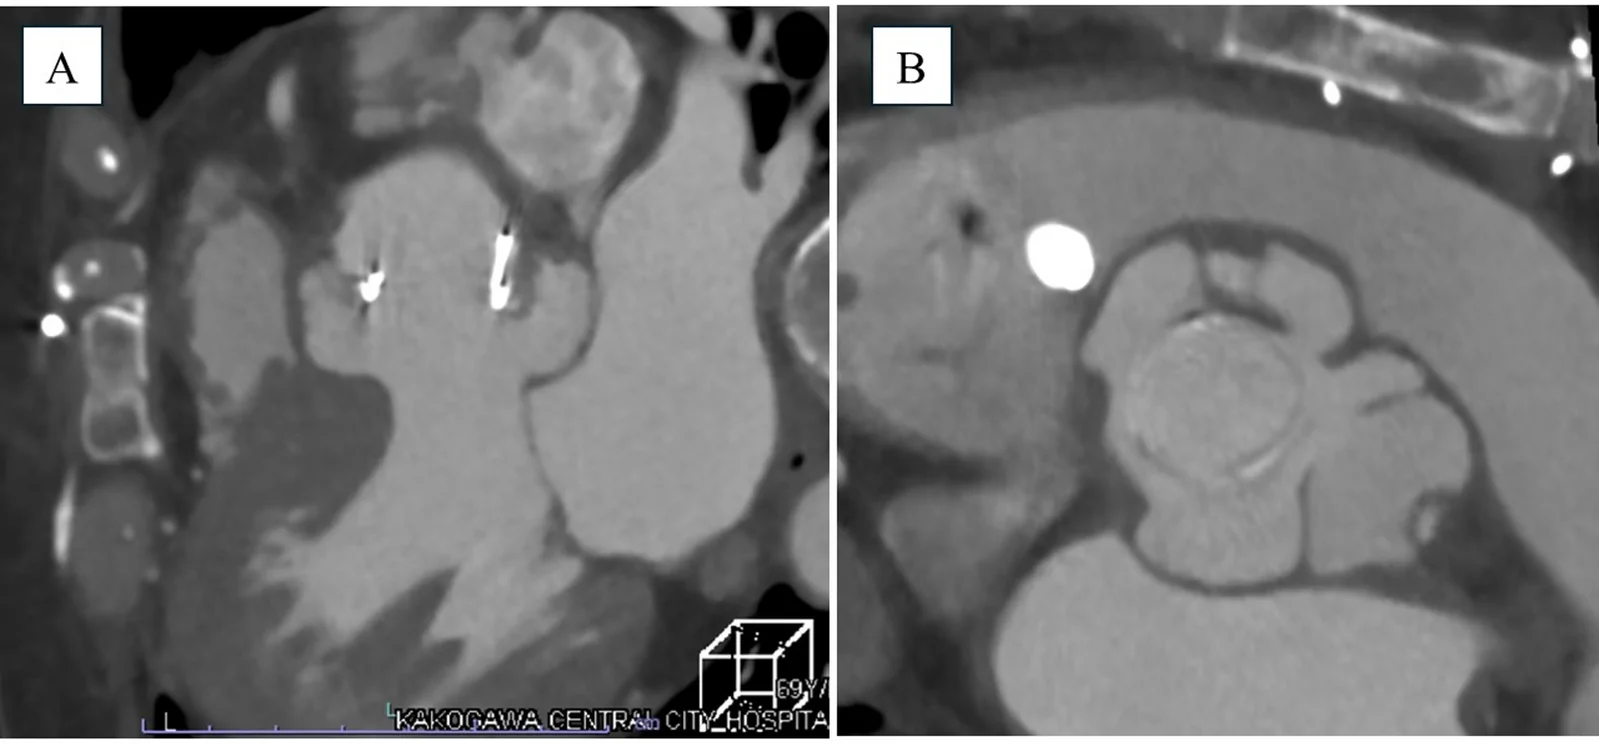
Preoperative contrast-enhanced computed tomography. A Long-axis view of the aortic root showing pseudoaneurysm formation at the annular level of the left and right coronary cusps. B Short-axis view perpendicular to the aortic annulus demonstrating pseudoaneurysm cavities surrounding the detached prosthetic valve
